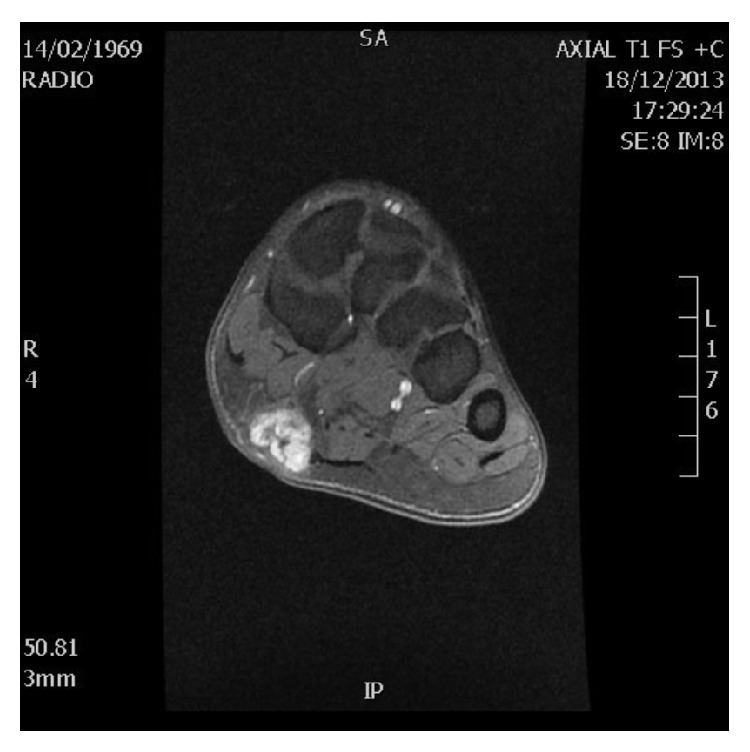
MRI of the right feet: axial T1-weighted image showing nodular soft-tissue masse sitting in the thickness of the distal part of the superficial medial plantar fascia and enhancing intensely Gadolinium.
